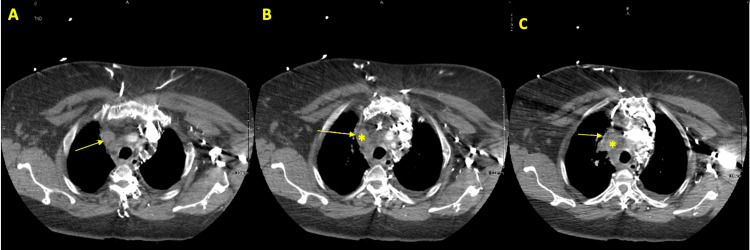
Transverse soft tissue CT slices. Transverse soft tissue CT slices showing the progressive compression of the SVC (arrow) by the mass (*, asterisk) (A->B->C). SVC: superior vena cava. CT: computed tomography.
